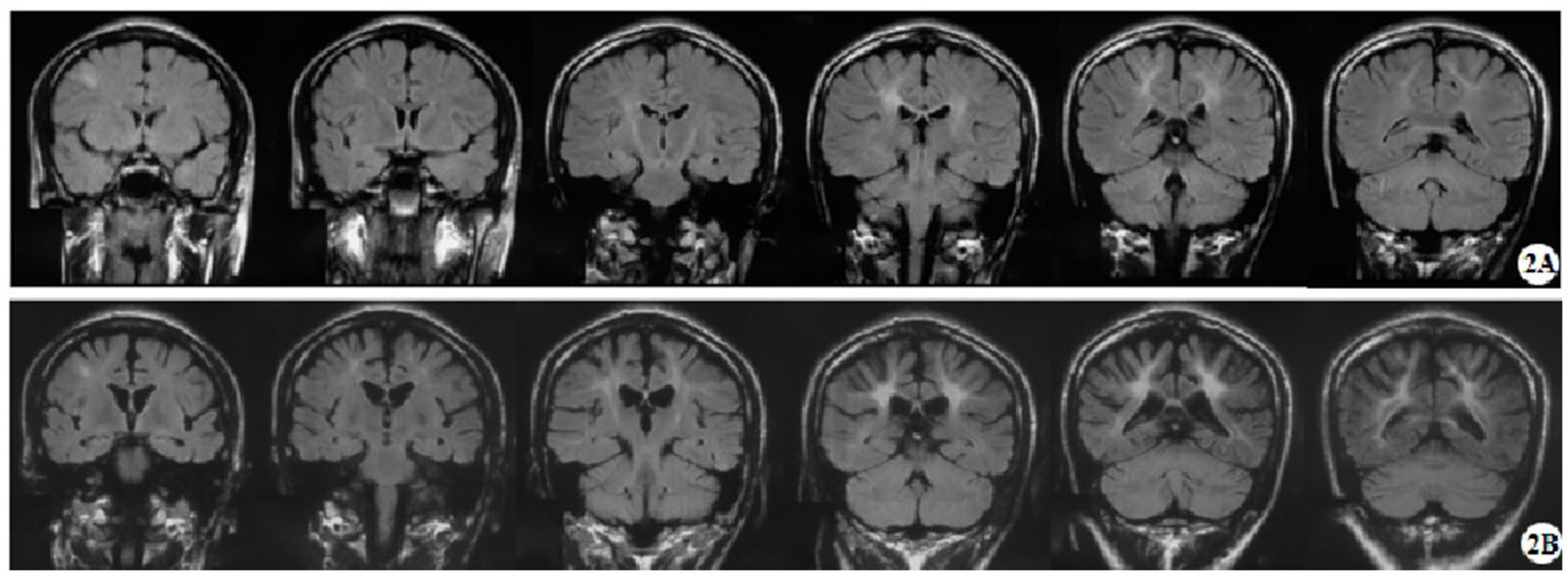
Representative brain MRI at the ages of 16 and 26 years (T2 FLAIR, coronal planes). (A) Brain MRI at the age of 16 detected symmetrical hyperintensities in the bilateral periventricular white matter and bilateral corticospinal tracts, as well as patchy hyperintensity in the right frontal subcortical region. (B) Brain MRI at the age of 26 detected symmetrical lesions in the bilateral corticospinal tracts, optic radiations, splenium of the corpus callosum, bilateral corona radiata, and the centrum semiovale (pre- and postcentral gyri) white matter, along with newly observed diffuse cerebral atrophy.
